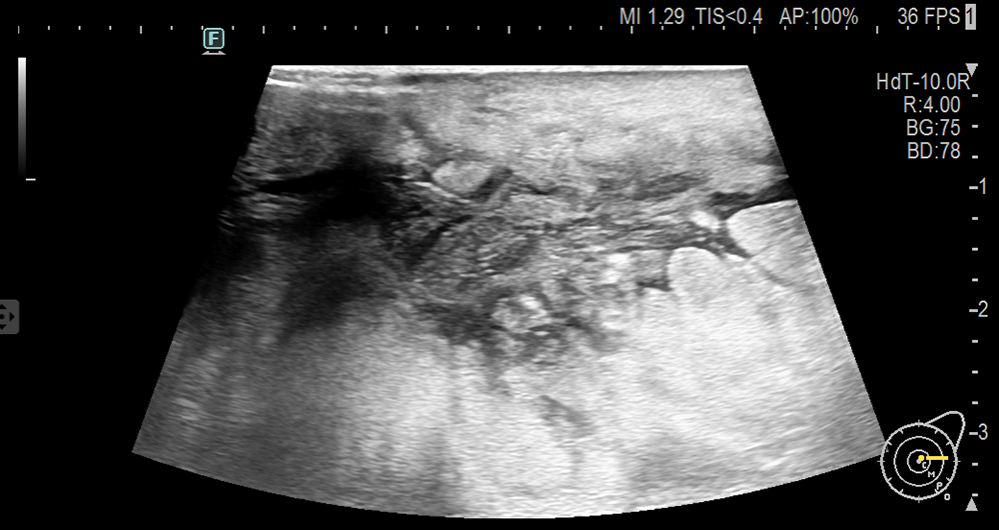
Ultrasound of breast tumor.
